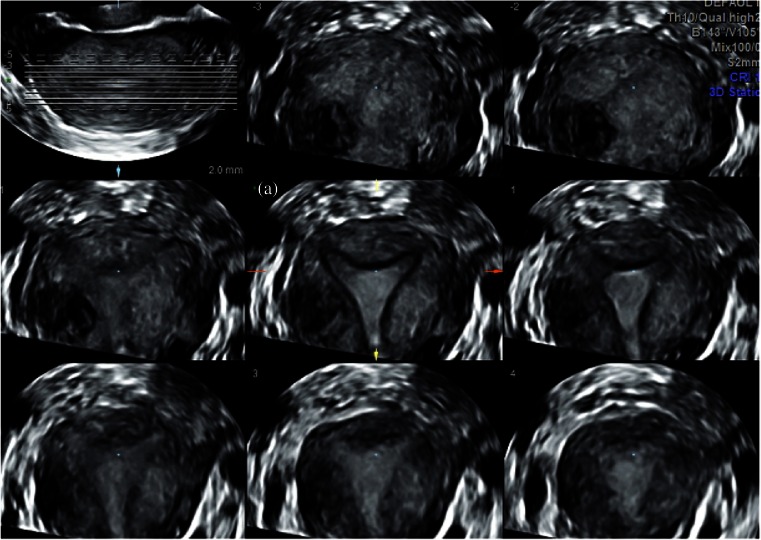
How to obtain an optimal 3D US coronal plane: tomographic ultrasound imaging (TUI) is the representation by a series of parallel slices through the volume and the distance between the slices as well as their number can be configured; the plane is optimal only if the slices or cutting line is exactly on the endometrium and the junctional zone at the level of the tubal ostia and isthmus (a) at the central plane
